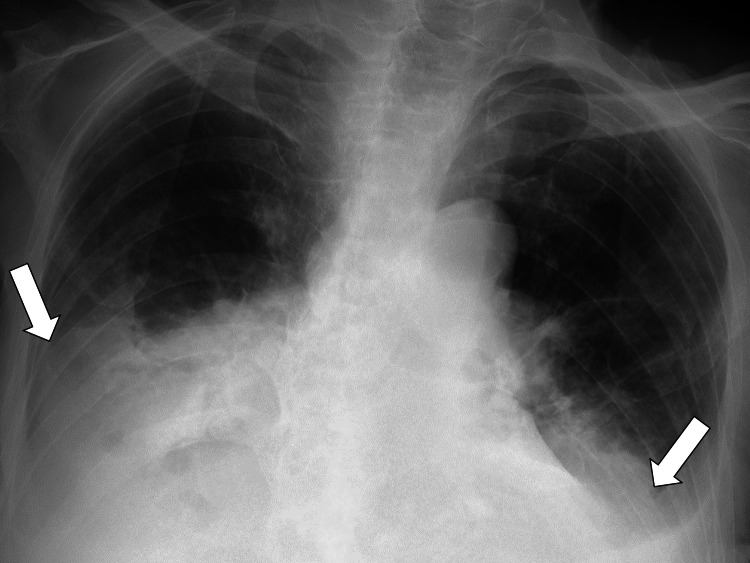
Chest X-ray at admission The bilateral costophrenic angles were dull.
